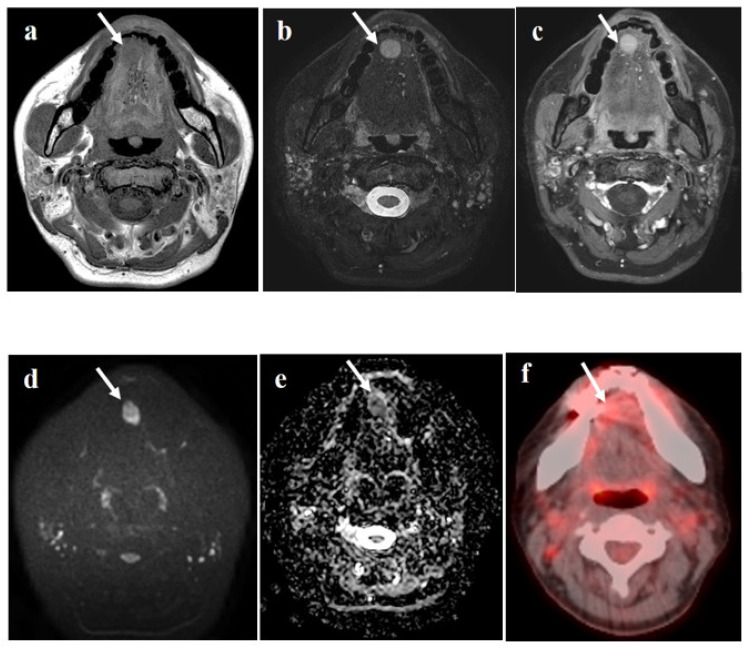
On T1-weighted image (a), the nodule is isointense with partially defined. On T2-weighted image (b), the nodule reveals omogeneously high signal intensity. The lesion shows a homogeneous enhancement on the contrast-enhanced T1-weighted image (c). The nodule showed diffusion restriction on diffusion-weighted image and ADC map (d,e). On 18-F FDG PET/CT image (f), the nodule shows mild FDG uptake with SUVmax of 4.8 g/mL.
